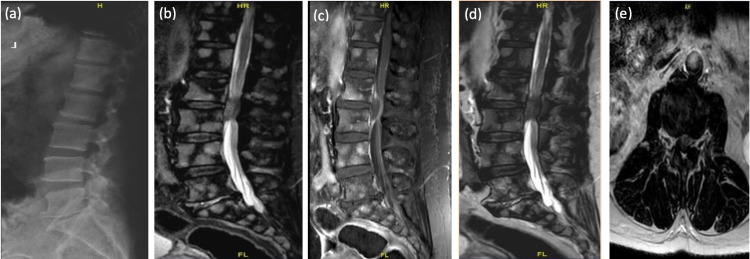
X-ray and lumbar MRI show preoperative changes. Preoperative x-ray of the lumbar spine showing degenerative changes without osseous abnormalities (a), sagittal STIR MRI (b), sagittal T1WI MRI without contrast (c) sagittal T2WI MRI without contrast (d), and axial T2WI MRI without contrast (e) demonstrating diffuse bone signals throughout the spine indicating neoplastic process, L2-L3 level anterior epidural soft tissue component with peripheral enhancement post-contrast causing significant compression of the thecal sac and cauda equina nerve roots.
